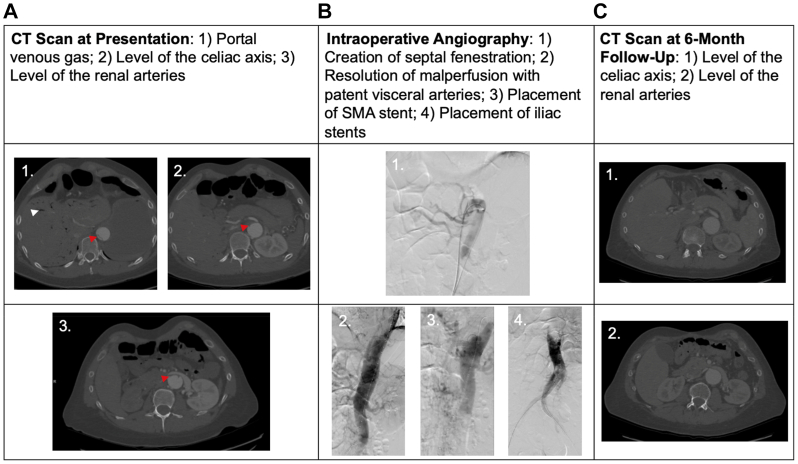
(A-C) Case 2. A 29-year-old man with Loeys-Dietz syndrome, prior type A aortic dissection (TAAD), and Society for Vascular Surgery/Society of Thoracic Surgeons (SVS/STS) type B_2,9_. *White arrow*, portal venous gas; *red arrow*, true lumen (TL). *CT*, Computed tomography; *SMA*, superior mesenteric artery.
